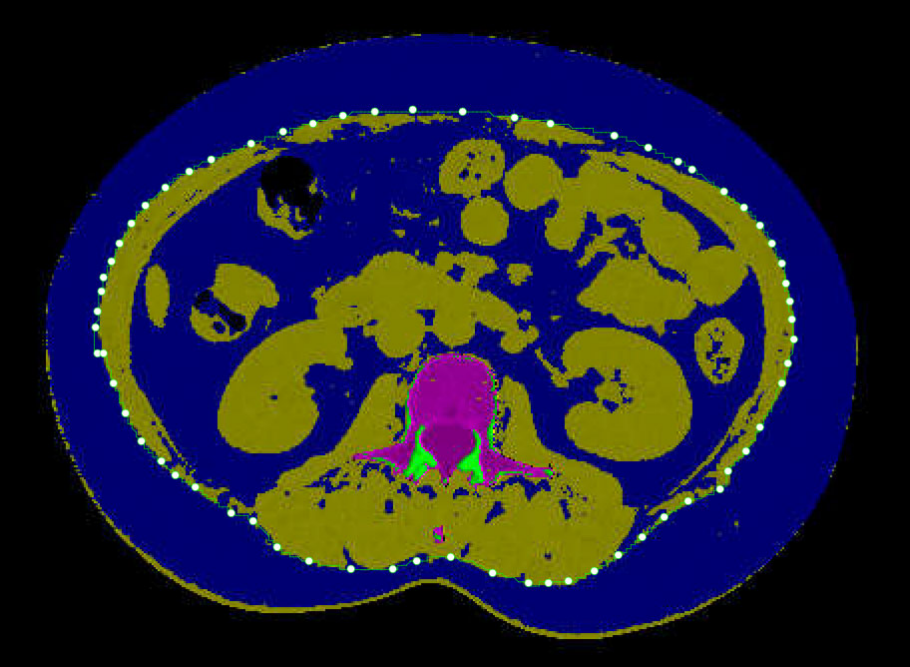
Abdominal quantitative computed tomography (QCT) image demonstrates abdominal fat distribution. The dark blue area represents non-adipose tissue and background, yellow regions indicate subcutaneous and visceral fat, and the magenta area represents specific visceral structures. The white dotted line delineates the boundary between subcutaneous and visceral fat compartments.
